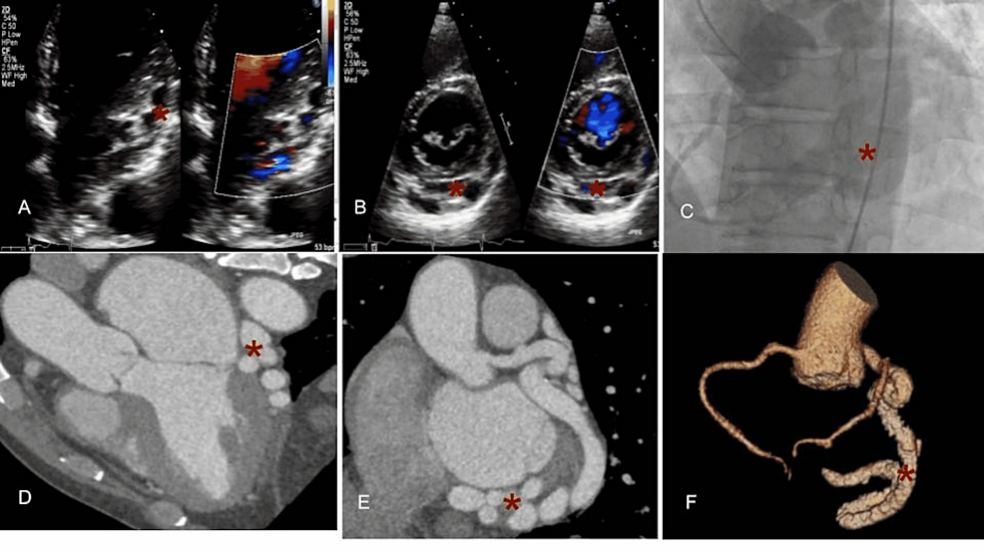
Multi-imaging modalities showing the coronary arteriovenous fistula [red asterisk*] Panel A:  Transthoracic echocardiogram apical four-chamber view showing dilated sinuses in the atrioventricular groove Panel B:  Transthoracic echocardiogram parasternal short axis view showing dilated sinuses along the inferior/inferolateral walls Panel C:  Aortic root angiogram filling the right and left coronary arterial system Panel D:  Computed tomography angiography of the heart showing sinus-like appearance and tortuous vessels in the atrioventricular groove Panel E:  Computed tomography angiography of the heart showing sinus-like appearance and tortuous vessels along inferior/inferolateral walls Panel F:  Computed tomography angiography of the heart 3D rendering showing the corresponding right and left coronary arteries along with dilated left circumflex artery arteriovenous fistula
